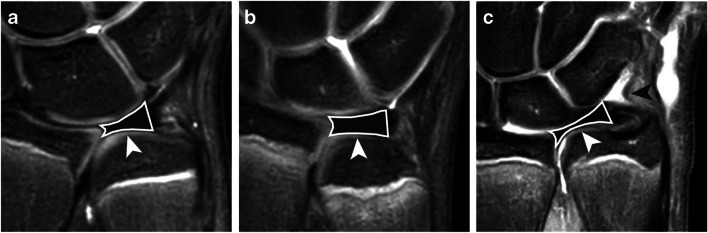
Coronal proton-density weighted fat-saturated MRI showing TFC morphology delineated in white and indicated by the white arrowheads a as a slightly radially tilted asymmetrical bowtie, b as a shorter, thicker and more horizontal structure, and c as a thinner and more stretched structure. Image c also shows the meniscus homolog morphology as a diffuse triangular shaped hypo-intensity (black arrowhead) directly lateral from the prestyloid recess
